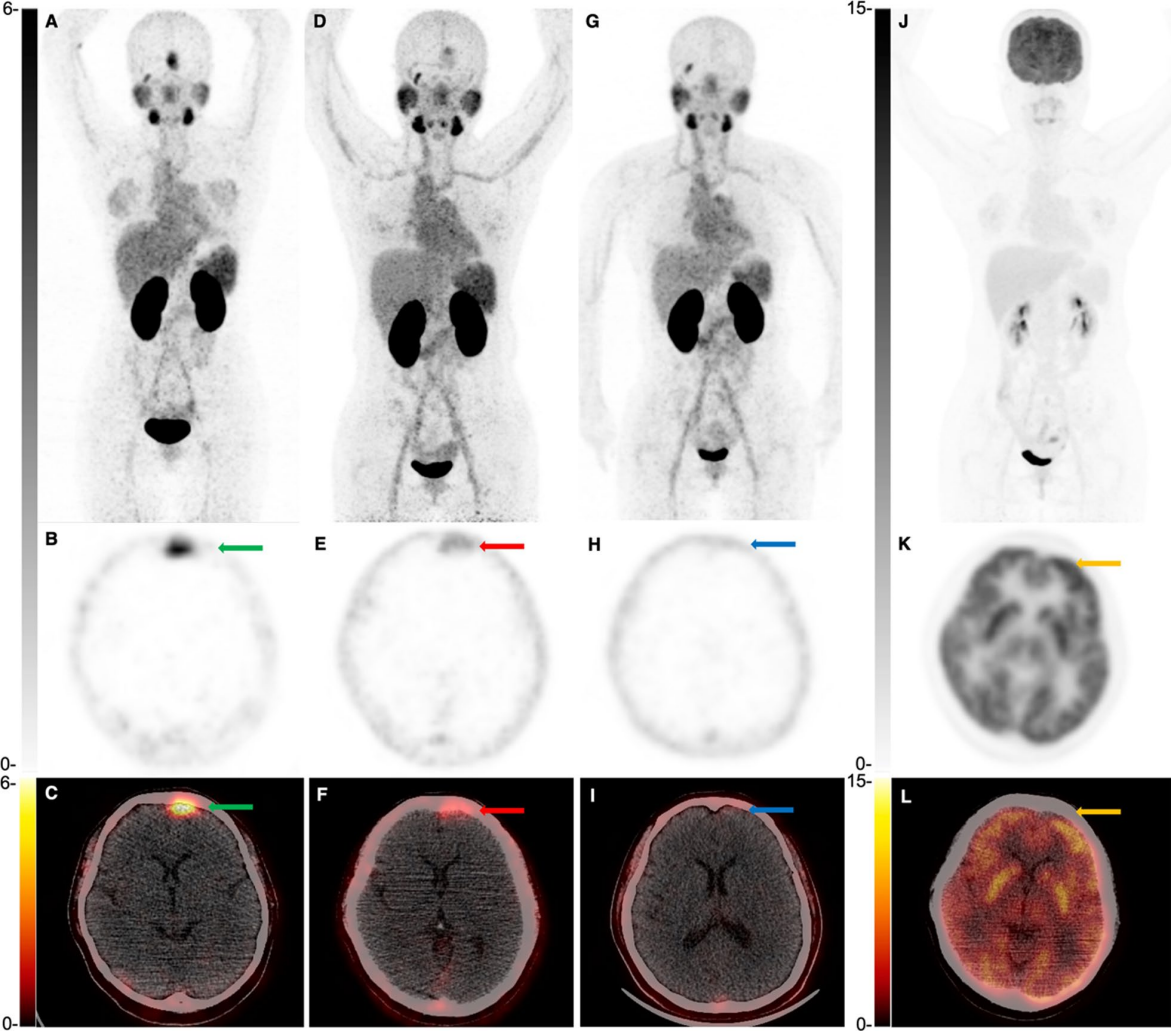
A 41-year-old female patient was diagnosed with a left frontal meningeal metastasis 20 months after surgical removal of the left lacrimal gland ACC. Pre-therapy ^68^ Ga-PSMA-617 PET/CT (A–C) demonstrated intense PSMA uptake of tumor (green arrow, SUVmax 7.0). ^68^ Ga-PSMA-617 PET/CT reexamination at 9 weeks after the 1st cycle of PSMA RLT (D–F) and 8 weeks after the 3rd cycle of PSMA RLT (G–I) revealed significantly decreased tracer uptake of tumor (red arrow, SUVmax 3.5; blue arrow, SUVmax 1.1), which reached the level of CR according to modified PERSIST criteria. However, ^18^F-FDG PET/CT (J–L) always exhibited no positive lesions (orange arrow)
